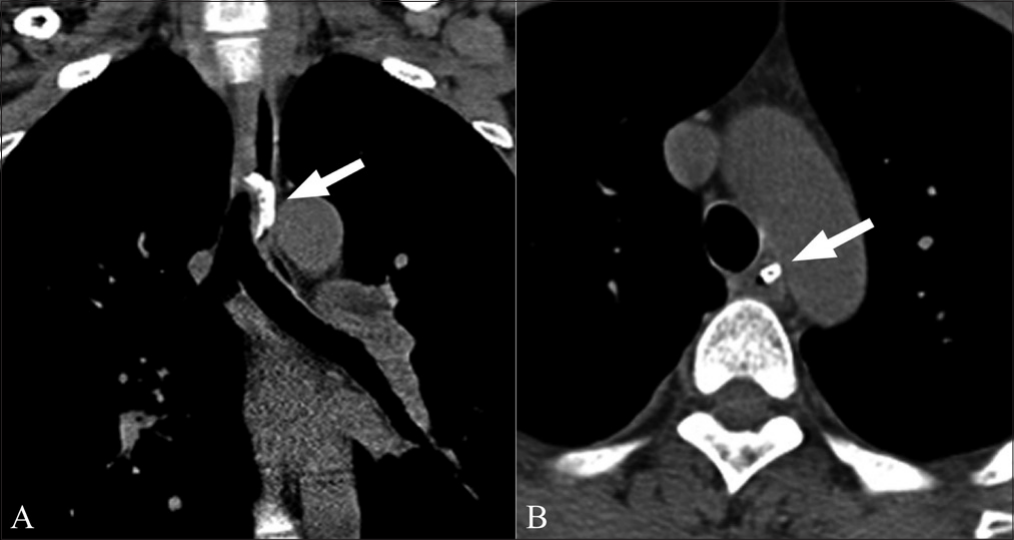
Coronal (A) and axial (B) noncontrast CT scans show a curvilinear FB at the level of the aortic arch (arrow). This is the second most common site of FB impaction within the esophagus. Note the close proximity to the aortic lumen which would partly explain the high incidence of vascular injuries within the mediastinum
